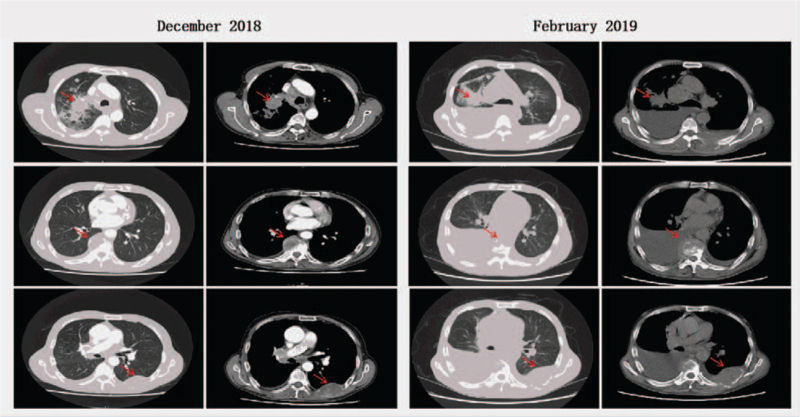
CT scans of the patient. The primary lesion and metastatic lymph nodes in the right lung had not shrunk, and the right pleural effusion had increased in size on the February 2019 scan compared with the previous scan. The curative effect was evaluated as disease progression. CT = computed tomography.
